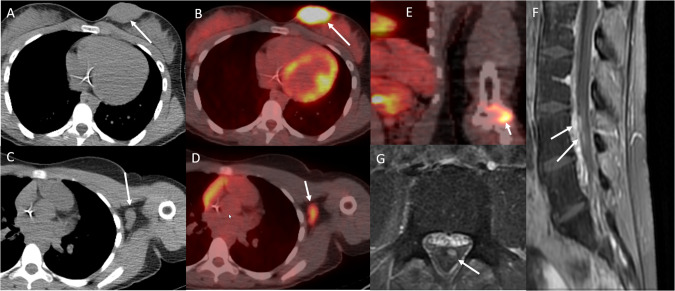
Radiologic Manifestations of BPDCN. Axial CT and fused PET/CT of a teenage female with BPDCN show nodular skin and subcutaneous soft tissue thickening in the left breast that is fluorodeoxyglucose-avid (arrows in A and B) and associated with fluorodeoxyglucose-avid left axillary lymphadenopathy (arrows in C and D) as well as a fluorodeoxyglucose-avid bone lesion in the left distal humerus (arrow in E). MRI of the spine shows enhancing nerve roots in the lumbar spinal canal, consistent with leptomeningeal disease (arrows in F and G).
